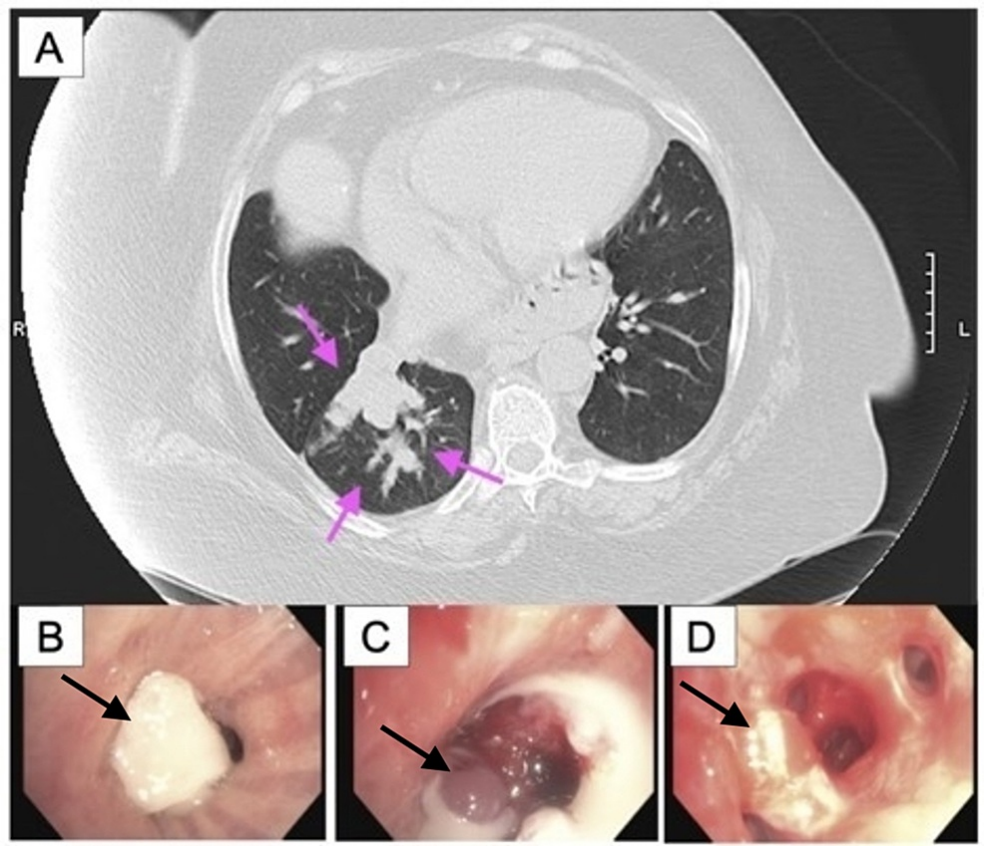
(A) CT showing an RLL consolidation with projecting opacities characteristic of a bronchocele. (B) Bronchoscopy showing proximal obstruction of the tumor at the level of the RLL by a bronchocele. (C) Evacuation of the mucous plug and pus formation with cryoprobe and suction revealing an obstructing endobronchial tumor. (D) An atypical carcinoid tumor with origin in the RLL medial base region following debulking with visualization of distal airways. RLL - right lower lobe
